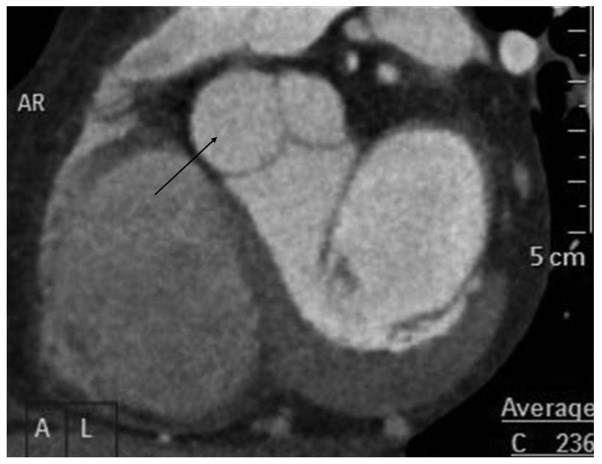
A 51-year-old female with an 8-year history of T2DM. Soft plaques were identified in the left descending coronary artery with an eccentric and unsmooth surface (black arrow). The CT value was 28 Hu. CT, computed tomography; T2DM, type 2 diabetes mellitus.
